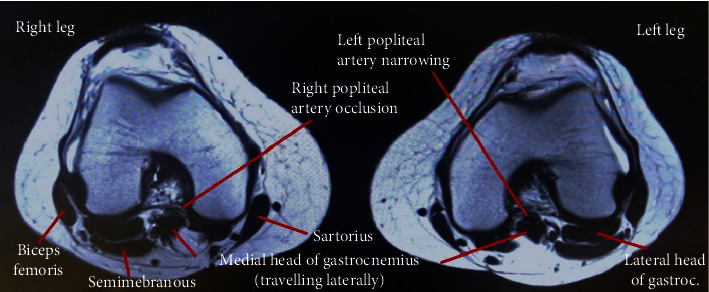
Magnetic resonance imaging demonstrating an axial view of bilateral knees. Note the lateral travel of the medial head of gastrocnemius, resulting in a right popliteal artery occlusion and left popliteal artery high-grade stenosis.
